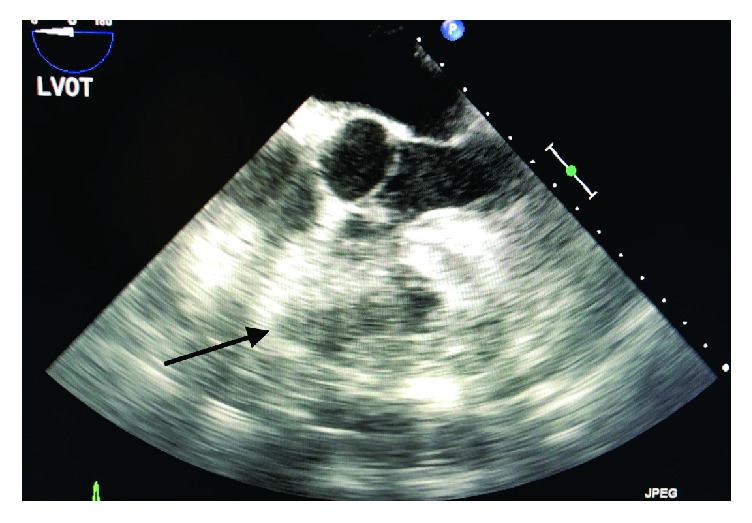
Transesophageal echocardiogram with ME 4CH view showing poor endocardial definition of the right ventricle and the thrombus.
